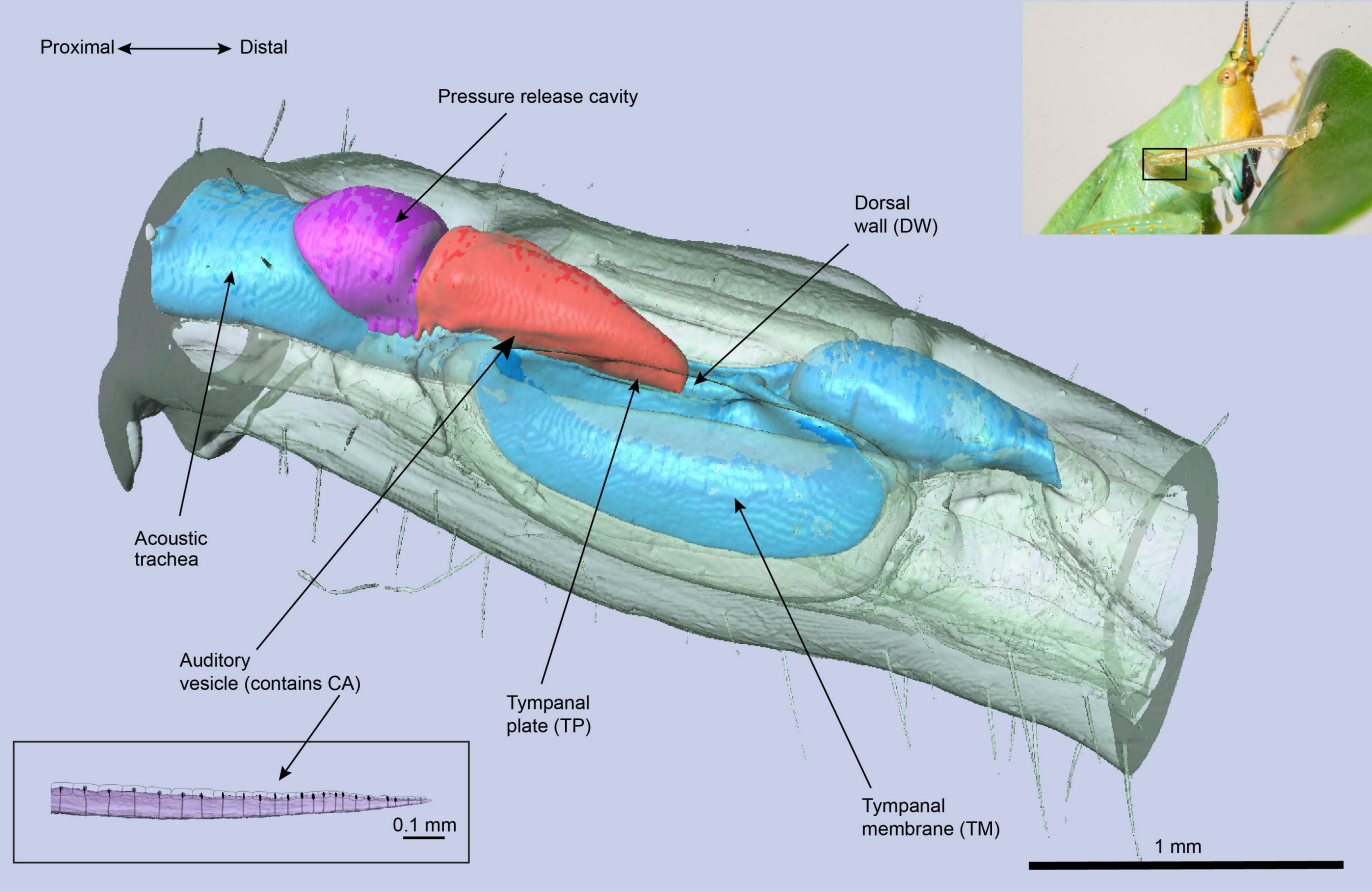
The *μ*-CT image of the *Copiphora gorgonensis* ear and its components.
